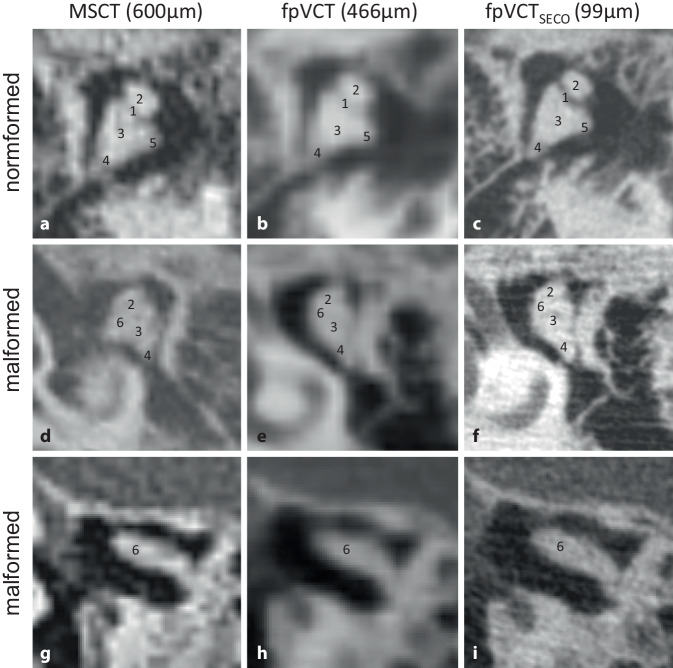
Visualization of the malleus–incus complex with different imaging modalities and settings. A representative image using a MSCT (600 µm slice thickness), b fpVCT (466 µm slice thickness), and c fpVCT_SECO_ (99 µm slice thickness). d–f Respective images demonstrating the malleus–incus complex with mild malformation and g–i with severe malformation. The following substructures of the malleus–incus complex were identified: *1* articulatio incudomalleolaris, *2* caput mallei, *3* corpus incudis, *4* crus breve incudis, *5* crus longum incudis, *6* fused art. incudomalleolaris. *MSCT* multislice computed tomography, *fpVCT* flat-panel volume computed tomography, *fpVCT*_*SECO*_ secondary reconstructions of fpVCT
